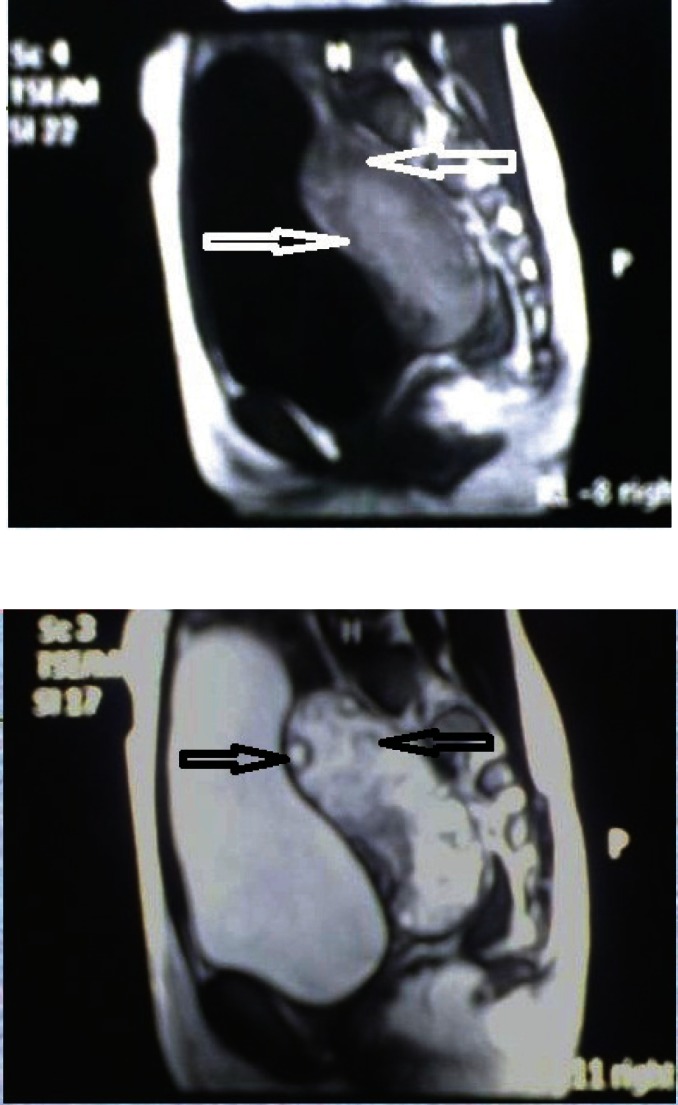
MRI of T1 & T2 weighted image isodense to hypointense capsule. The image did not show any fat component within and multiple normal ovarian follicles(arrows) noted in the periphery of the mass.
